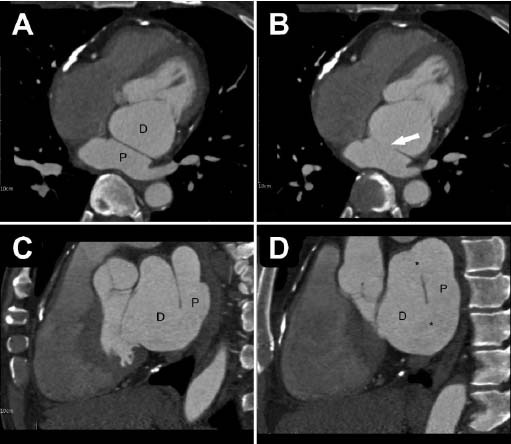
(A, B) The axial view of the cardiac computed tomography angiography revealed an accessory septum between the proximal chamber (P) and the distal chamber (D) in the left atrium. (B, C, D) Large openings in the accessory septum were noted in the axial and sagittal views. (white arrow and the asterisk).
